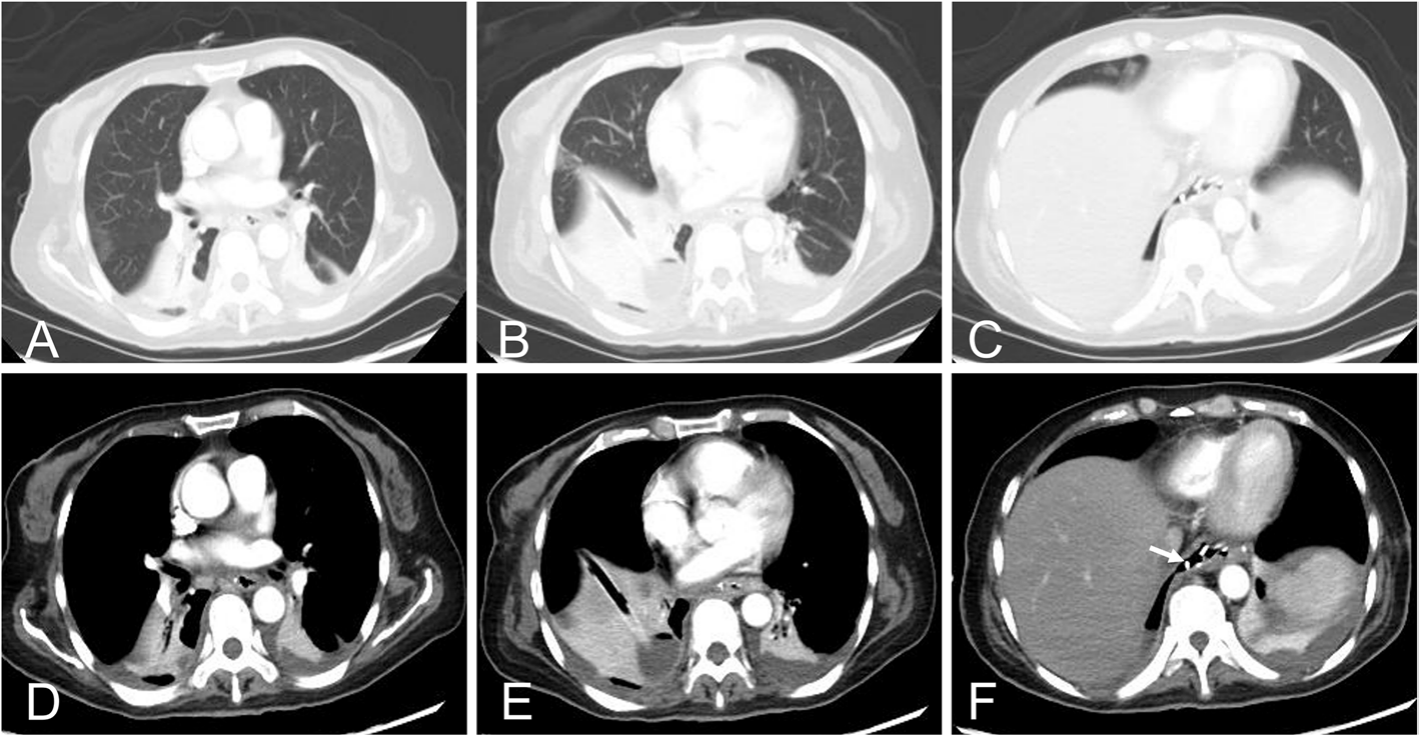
A 56-year-old female who underwent total gastrectomy and esophagojejunostomy for gastric cancer developed fever and chest tightness 4 days after surgery. CT examination revealed communication between the esophagojejunal anastomosis and the right thoracic cavity (white arrow), with inflammation, atelectasis of the lung, and fluid accumulation in the pleural space
